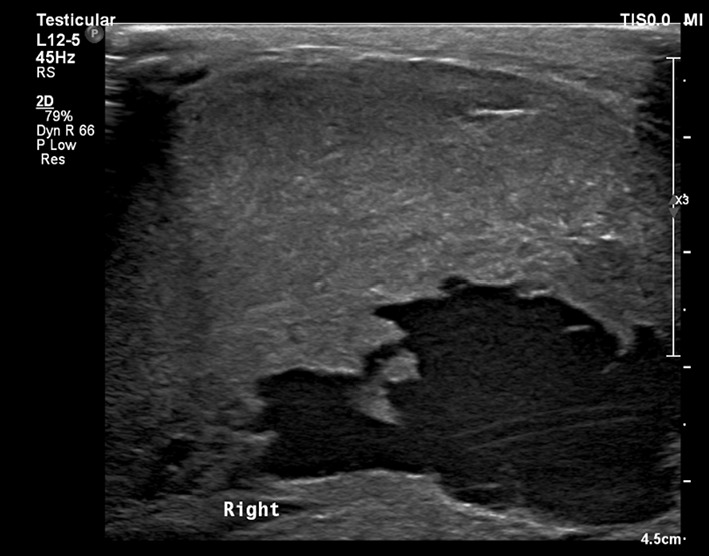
Ultrasound of right testicle.
